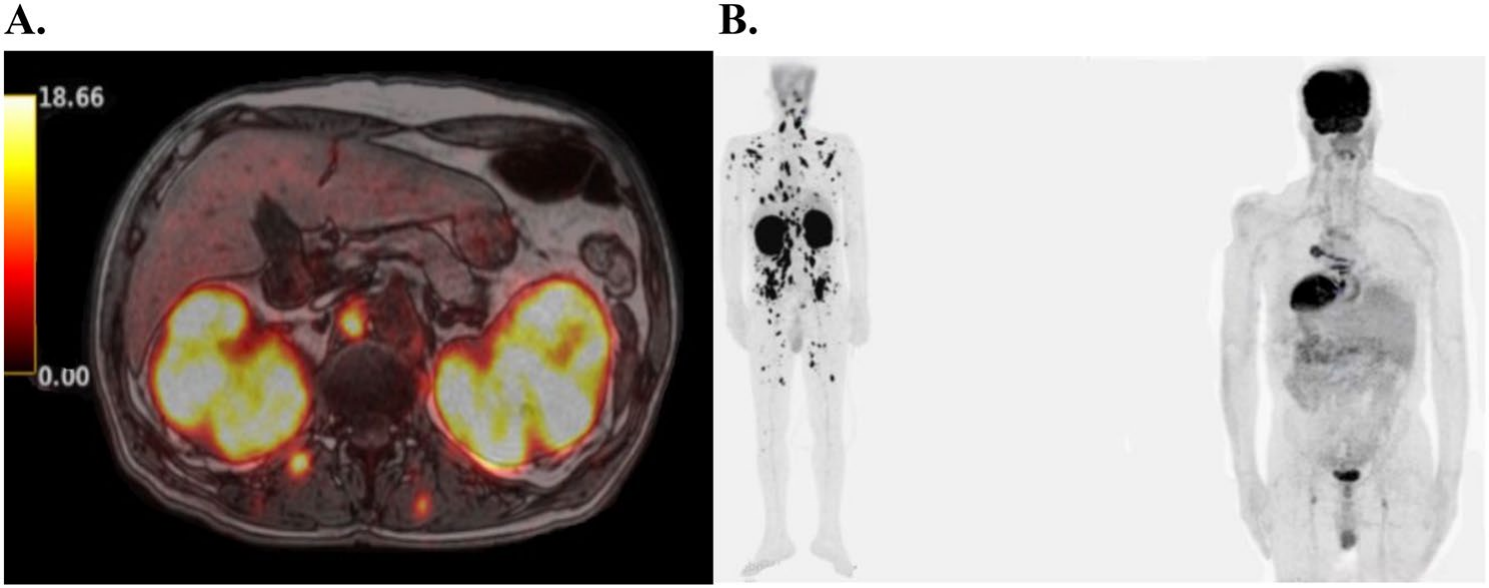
(A) Axial section PET/MR imaging at the T12 vertebral level demonstrates lobulated contours with bilateral renal involvement. Focal increased ^18^F-FDG uptake in the kidneys and surrounding soft tissues (B) The pre-treatment Maximum Intensity Projection (MIP) image demonstrates nodular/diffuse patterns of increased ^18^F-FDG uptake in the trunk, proximal extremities, and bilateral kidneys. The post-treatment MIP image revealed that the distribution of the radiopharmaceutical in all other visualized body regions and bone structures was consistent with physiological distribution
